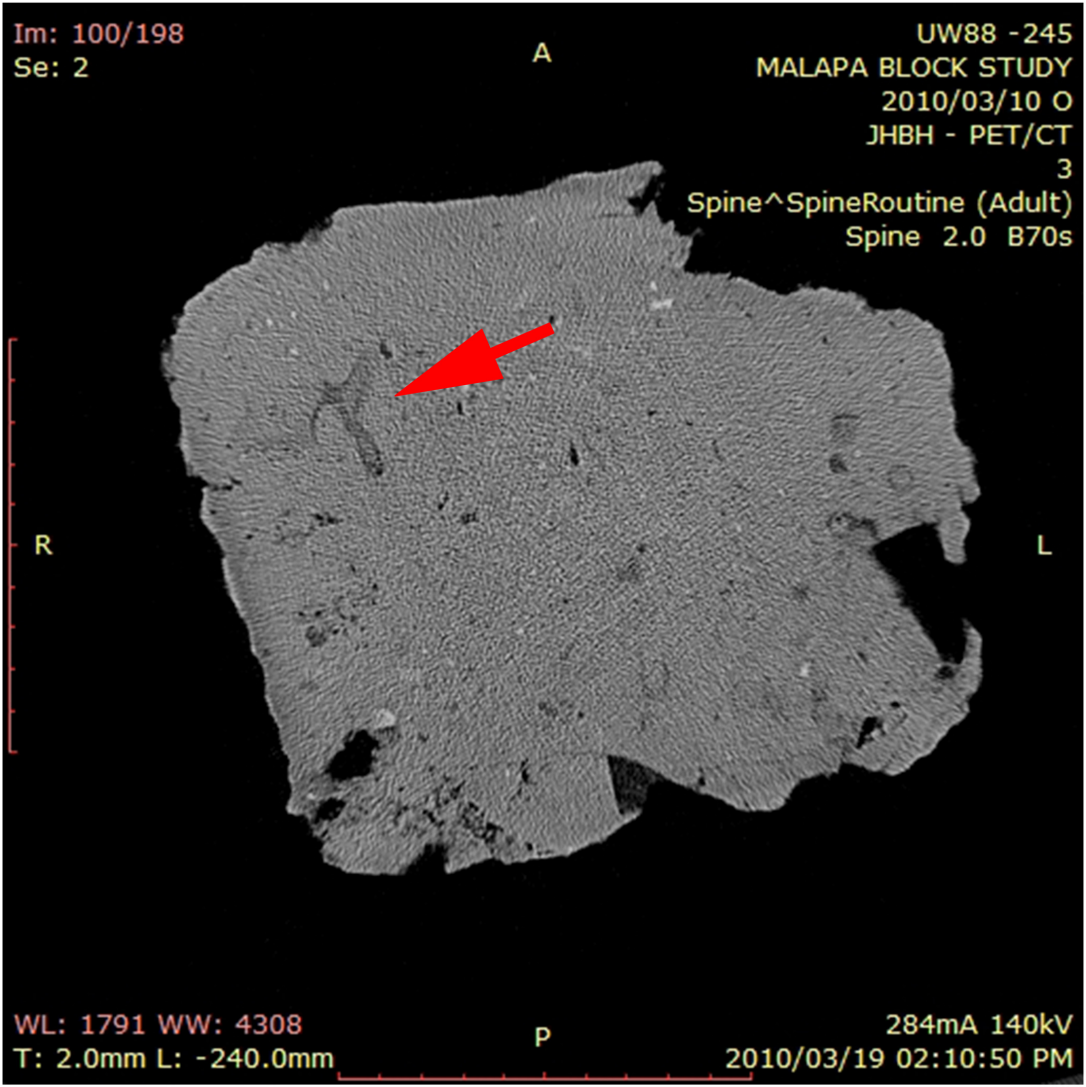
B 245 (UW 88–152)—Hominin vertebra identified on CT (arrow). Seen in sagittal projection.
